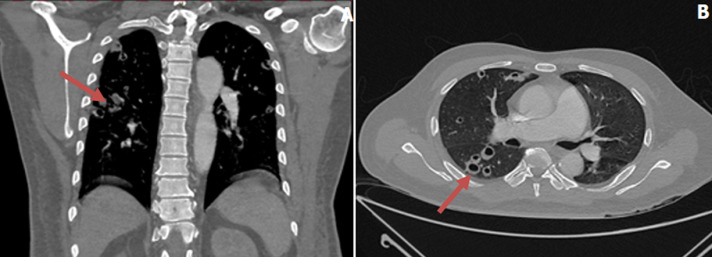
A, B) CT chest (coronal and axial views): multiple parenchymal and pulmonary nodular lesions, some with air-fluid levels (abscesses), indicated by blue arrows
